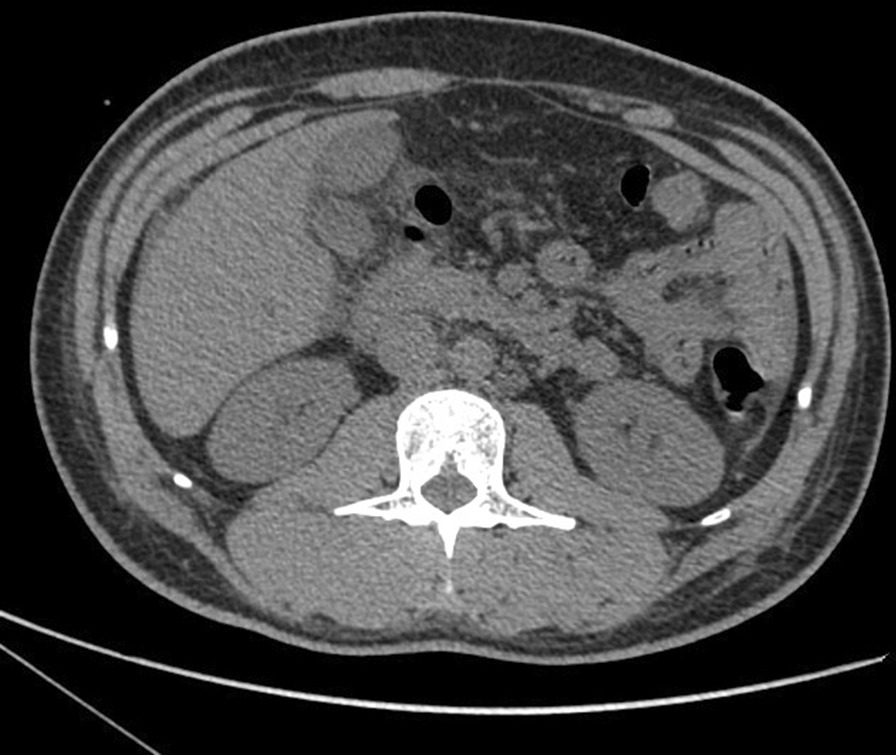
Abdominal computed tomography. The gallbladder wall was edematous and thickened, and no gallstone was observed
